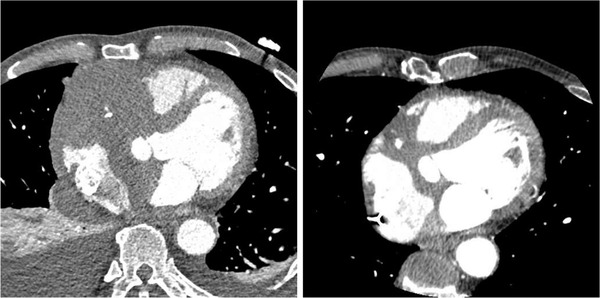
Computed tomography images of the chest, showing extensive lesions in the right atrioventricular septum before (left) and after (right) the first line of chemotherapy, with artifacts due to the pacemaker
